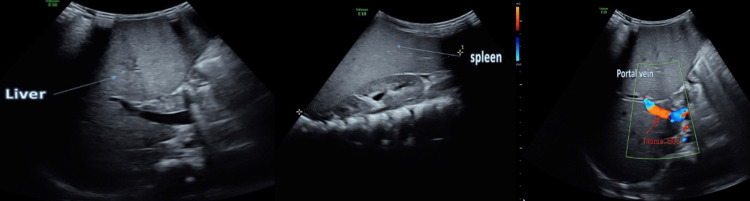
Doppler ultrasound reports hepatosplenomegaly.
